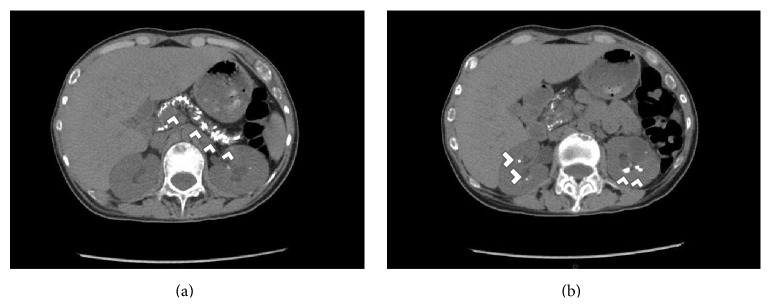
Abdominal computed tomography (CT) on admission. (a) Diffuse pancreatic calcifications; (b) bilateral kidney calcifications on axial computed tomography. Findings are marked with white arrow heads.
